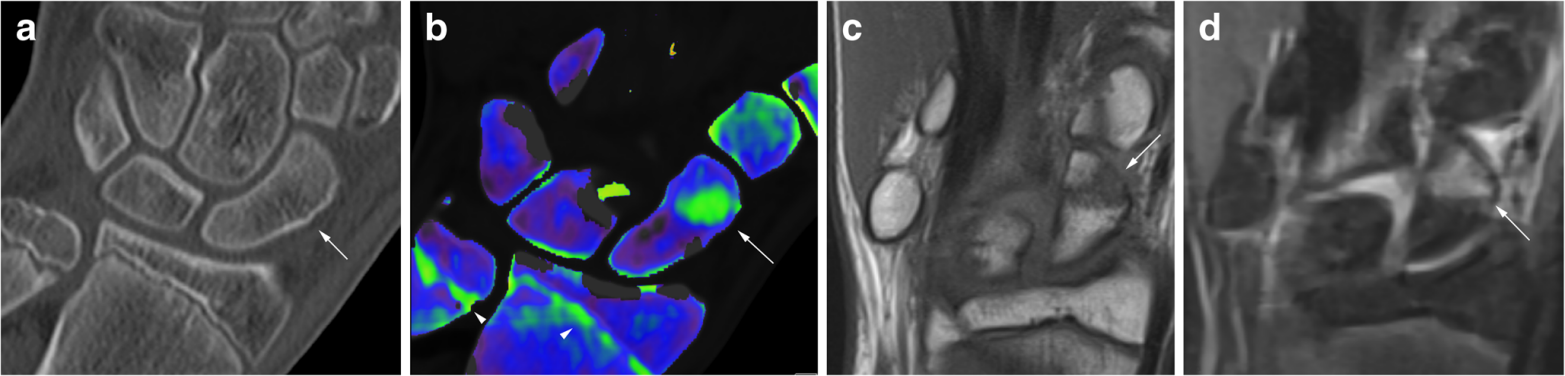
A 15-year-old male with a non-displaced scaphoid fracture shown as a subtle line on CT coronal reconstruction with 2 mm slice thickness (Fig.2a) and findings consistent with BME on the coronal VNCa image (Fig. 2b), color coded in green (arrow). Note the epiphyseal line on the distal radius, and the ulna is also color coded in green (arrowhead). MRI with coronal T1-weighted image (Fig. 2c) and coronal fluid-sensitive sequence (Fig. 2d) confirm BME
